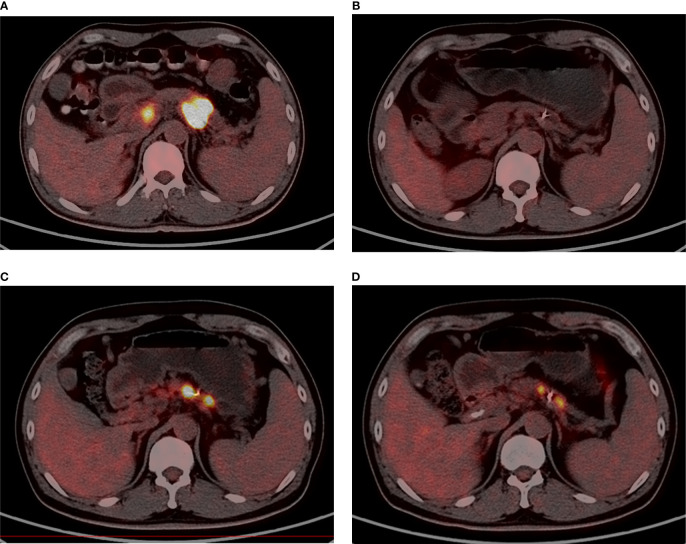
A male, 47 years old, who has adenocarcinoma, with stage III before the first SBRT [(A); SUVmax = 23.2]. Nine months after the first SBRT (35 Gy/7Fx), the tumor had shrunk drastically [(B); SUVmax = 8.0]. The patient relapsed at 22 months after the first SBRT and underwent re-irradiation [(C); SUVmax = 21.8]. Four months after re-irradiation (32 Gy/8Fx), the SUV value declined [(D); SUVmax = 9.2].
